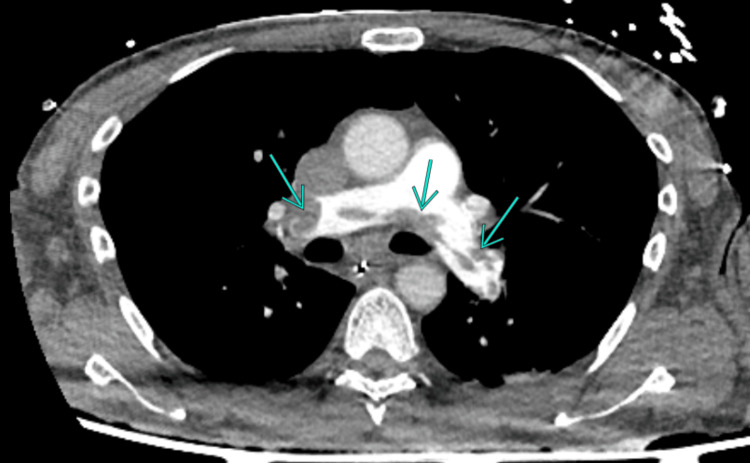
CTPA showing bilateral filling defects consistent with thrombi in the pulmonary arteries CTPA, CT pulmonary angiography
